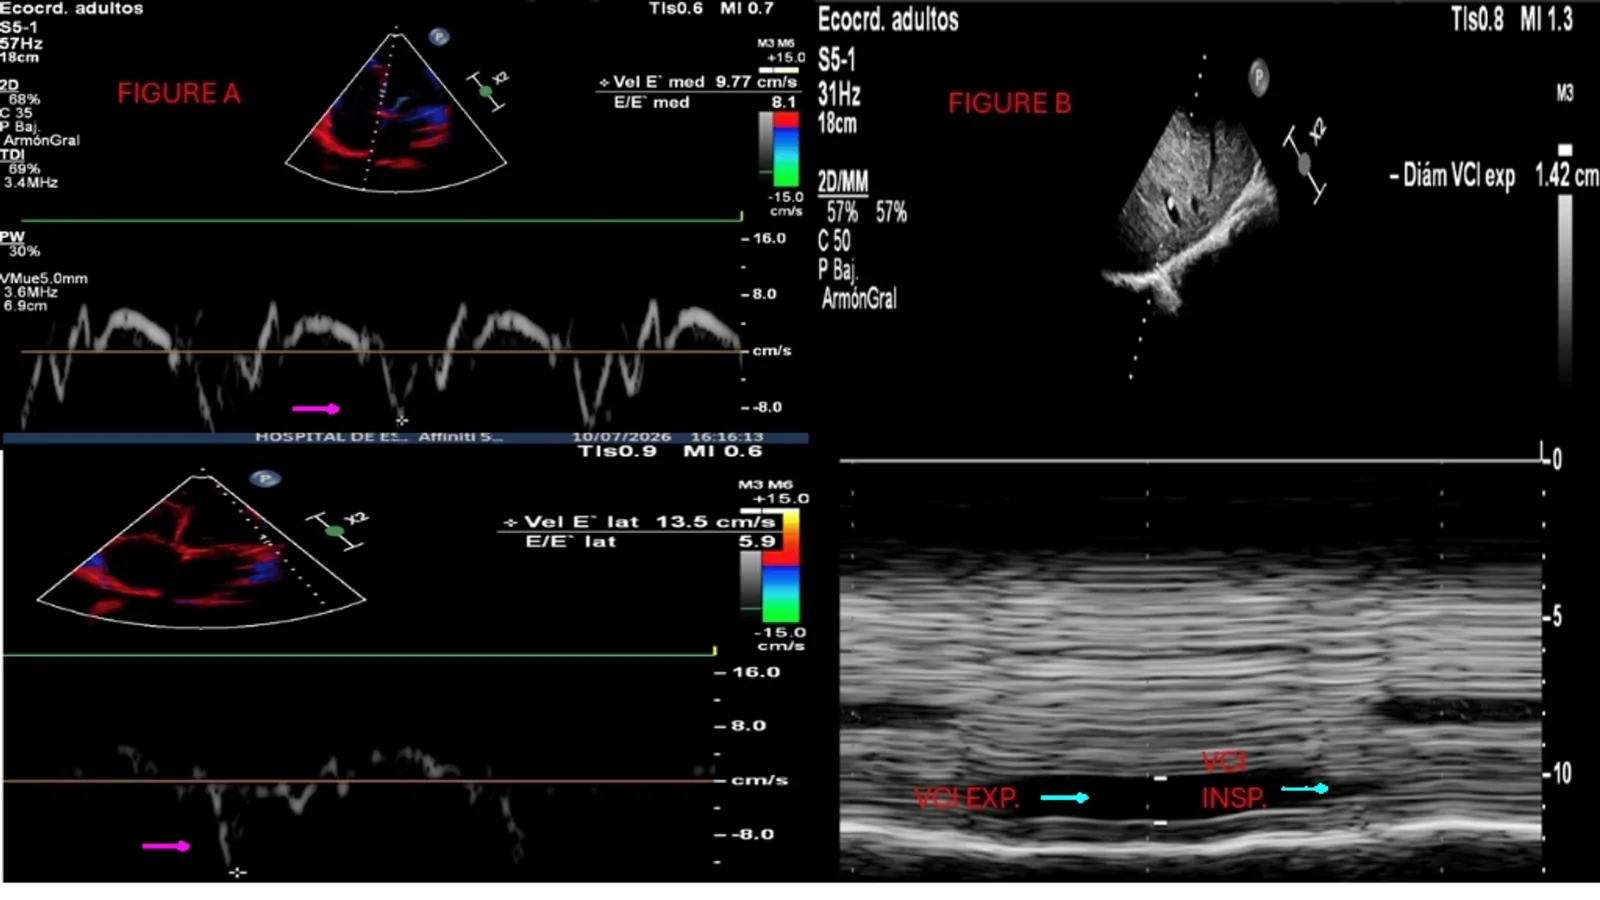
Functional echocardiographic resolution during outpatient follow-up (A) M-mode and tissue Doppler: The magenta arrows show resolution of the septal bounce and normalized annular velocities (lateral E' > septal E'). (B) Subcostal view: The cyan arrows indicate the diameter of the inferior vena cava during expiration (vena cava inferior (VCI) exp.) and its normalized >50% inspiratory collapse (VCI insp.).
